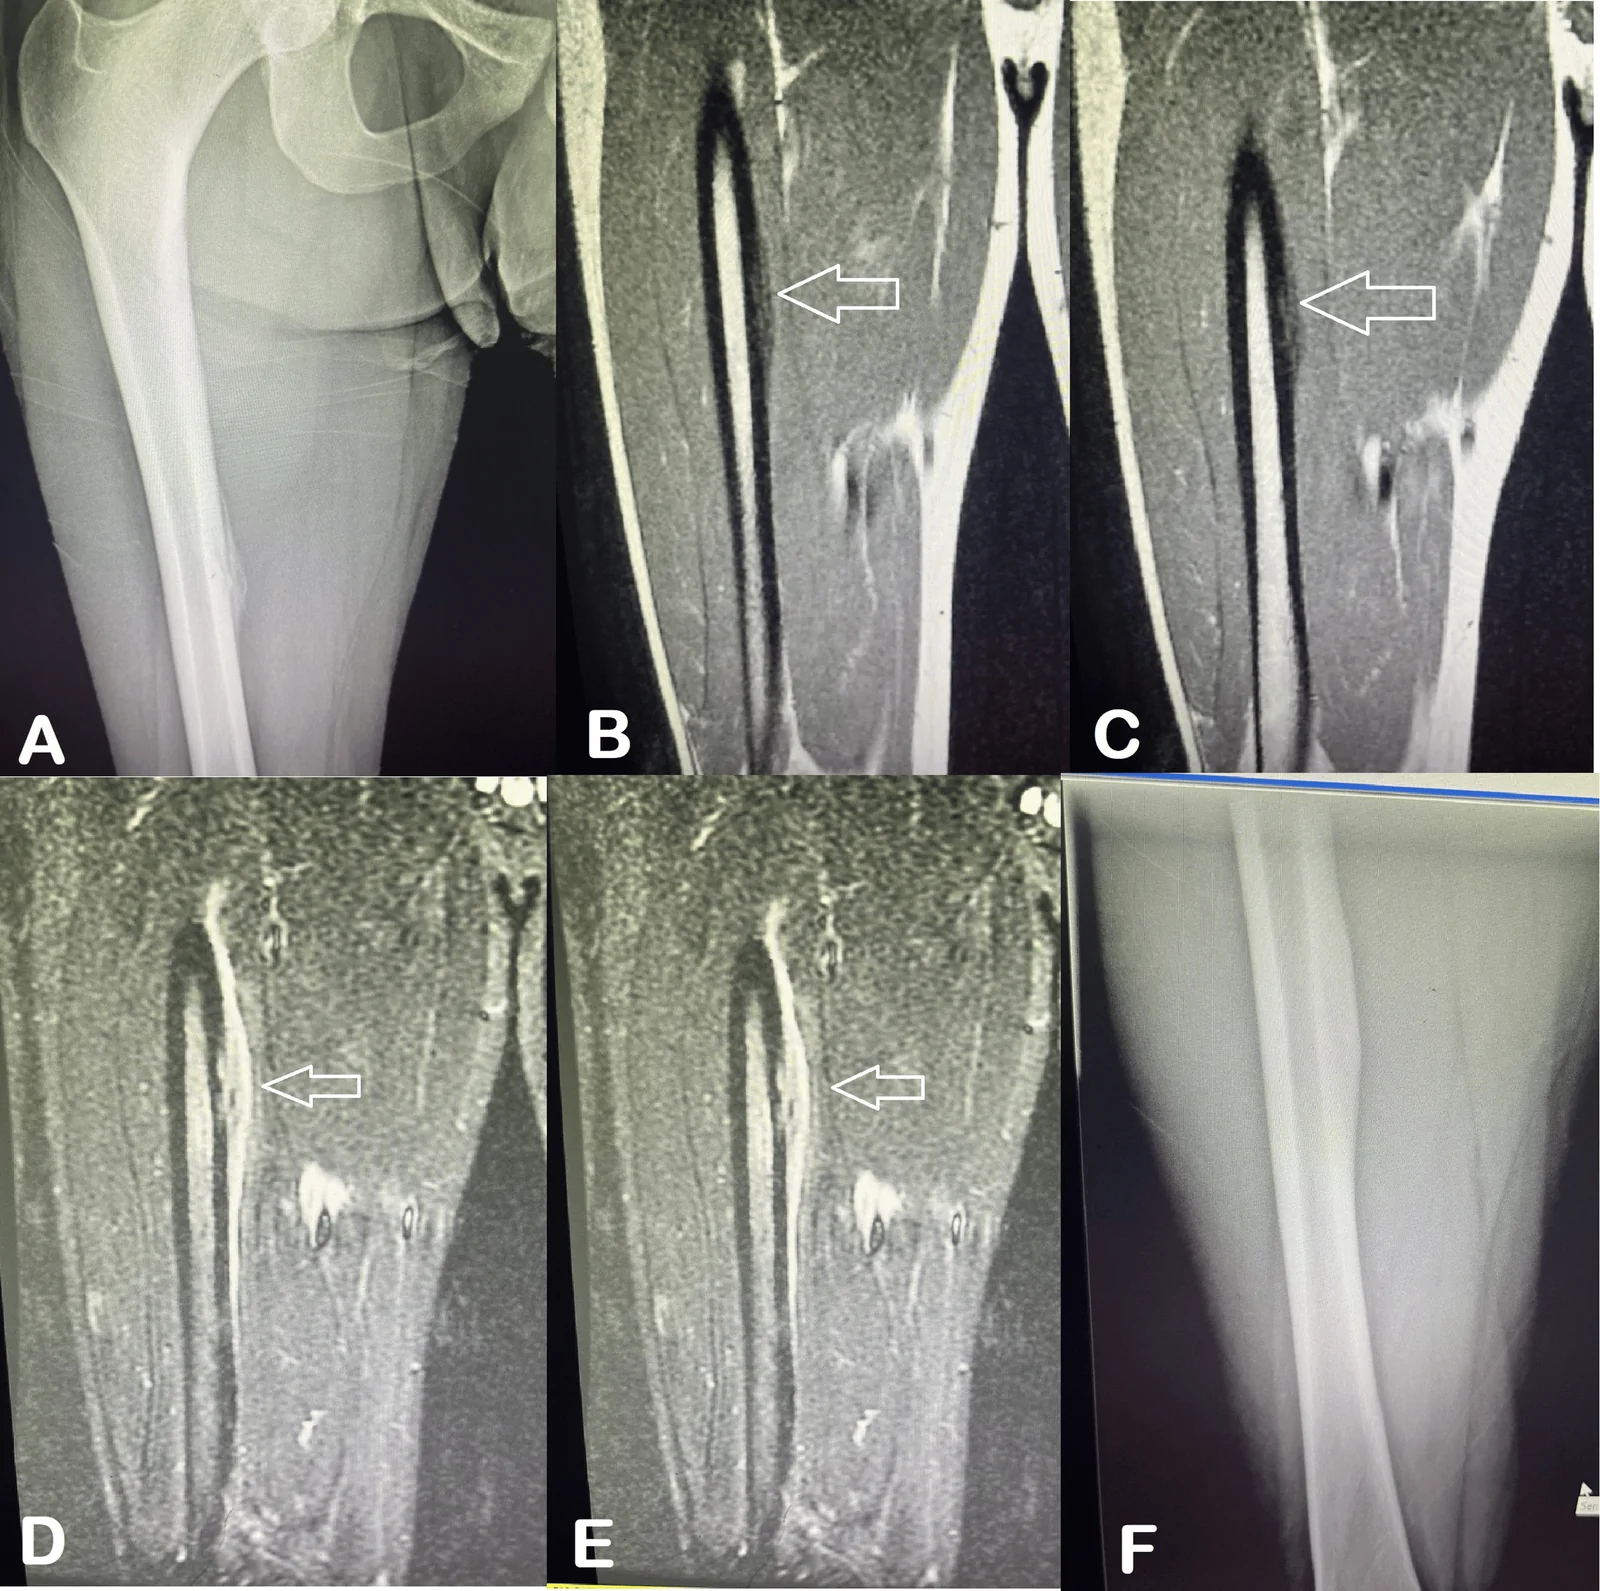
Femur radiograph of a 19-year-old male (A) AP femur radiograph obtained at the initial presentation of a new 19-year-old male military recruit complaining of right thigh pain, showing an incomplete stress fracture of the femur shaft on the compression side with callus formation. (B, C) T1 coronal thigh MRI images of the same patient showing periosteal reaction on the compression side of the femur without an obvious fracture line (arrow). (D, E) T2 coronal thigh MRI images showing obvious periosteal reaction with bone edema on the compression side of the femur shaft representing a femur stress fracture (arrow). (F) Three-month follow-up AP femur radiograph of the same patient showing complete healing after conservative treatment with complete resolution of symptoms. AP, anteroposterior.
